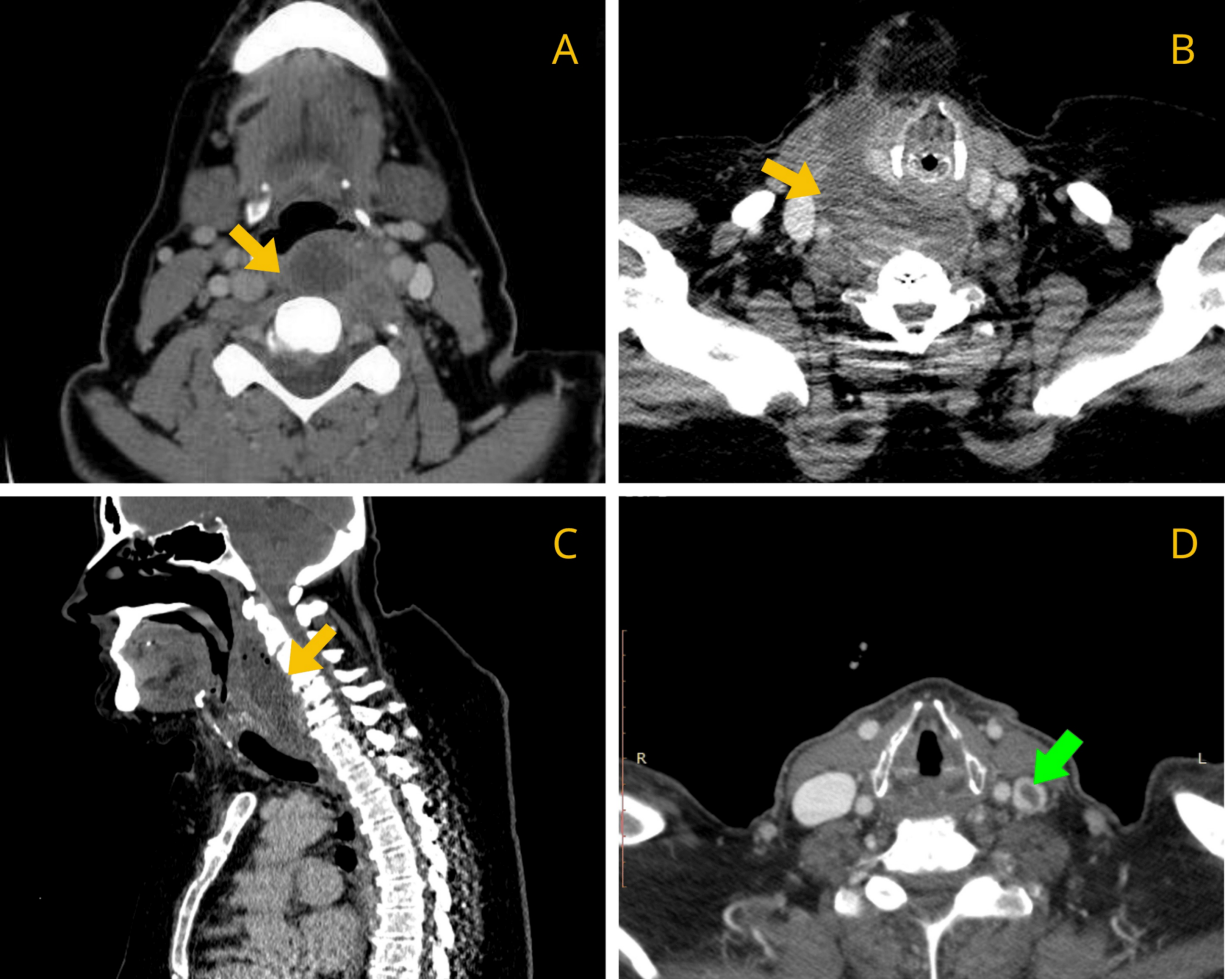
Contrast-enhanced CT images of patients 7 (A), 8 (B-C) and 10 (D). (A) Abscess extending to the prevertebral space (yellow arrow), (B-C) Abscess extending to the prevertebral space, posterior pharyngeal space, danger space (yellow arrow), (D) Left jugular vein thrombosis (green arrow).
